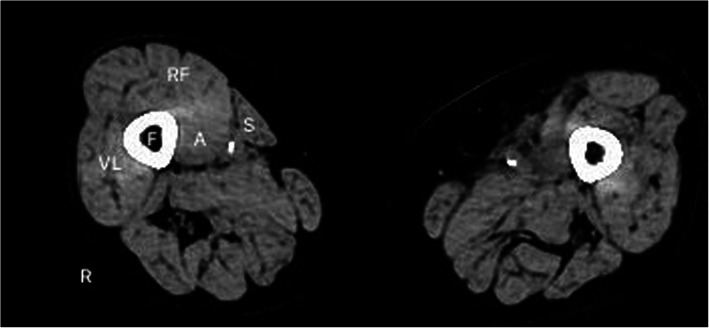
CT cross-section image at the level of the upper thigh reveals atrophic changes of the rectus femoris, adductor muscle, and sartorius muscle on the left. Normal side is labeled for identification of structures:S = sartorius muscle, A = adductor muscle, RF = rectus femoris, VL = vastus lateralis, F = femur
